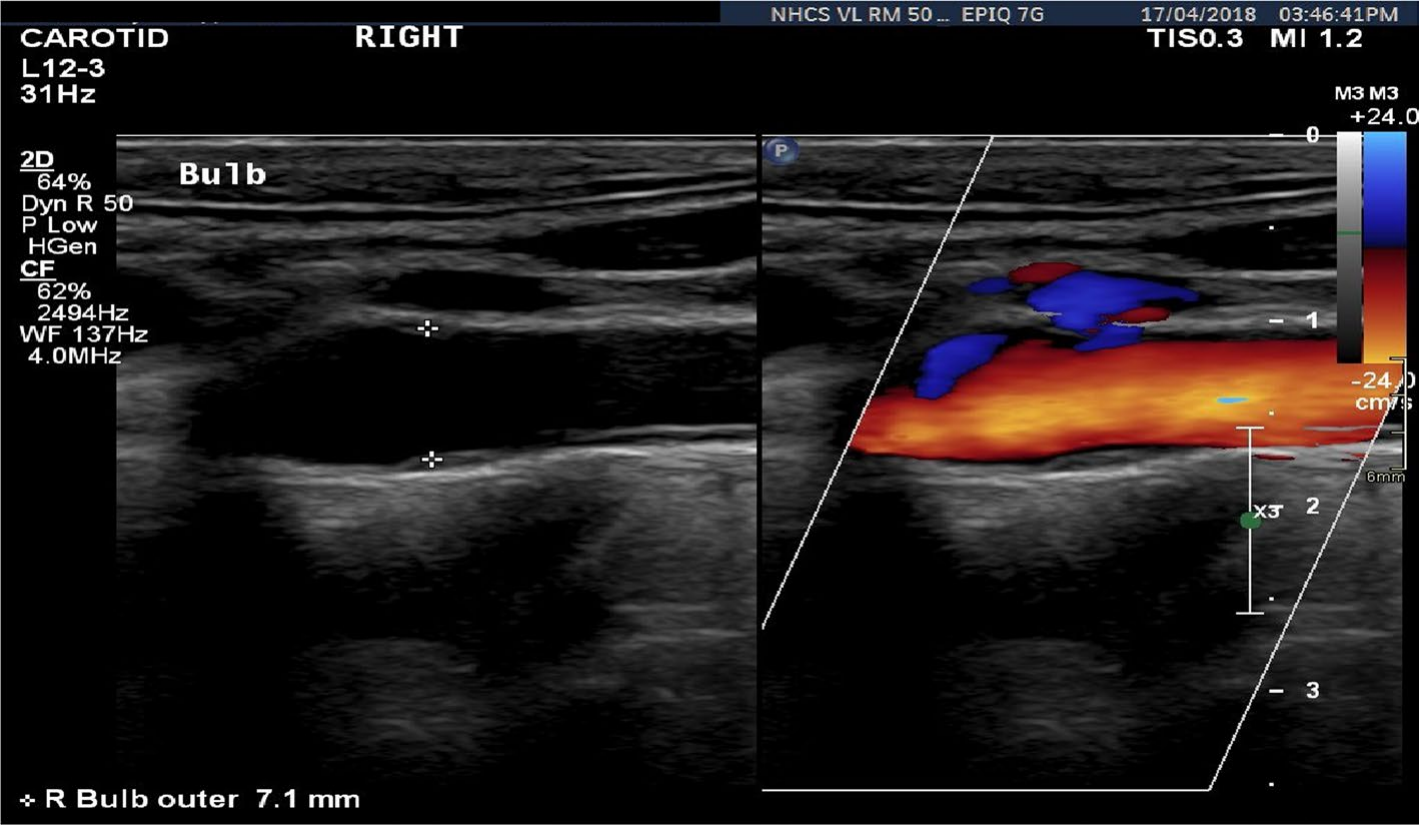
A duplex ultrasound output displaying the B-mode and colour flow doppler image side-by-side (credits: National Heart Center Singapore)
